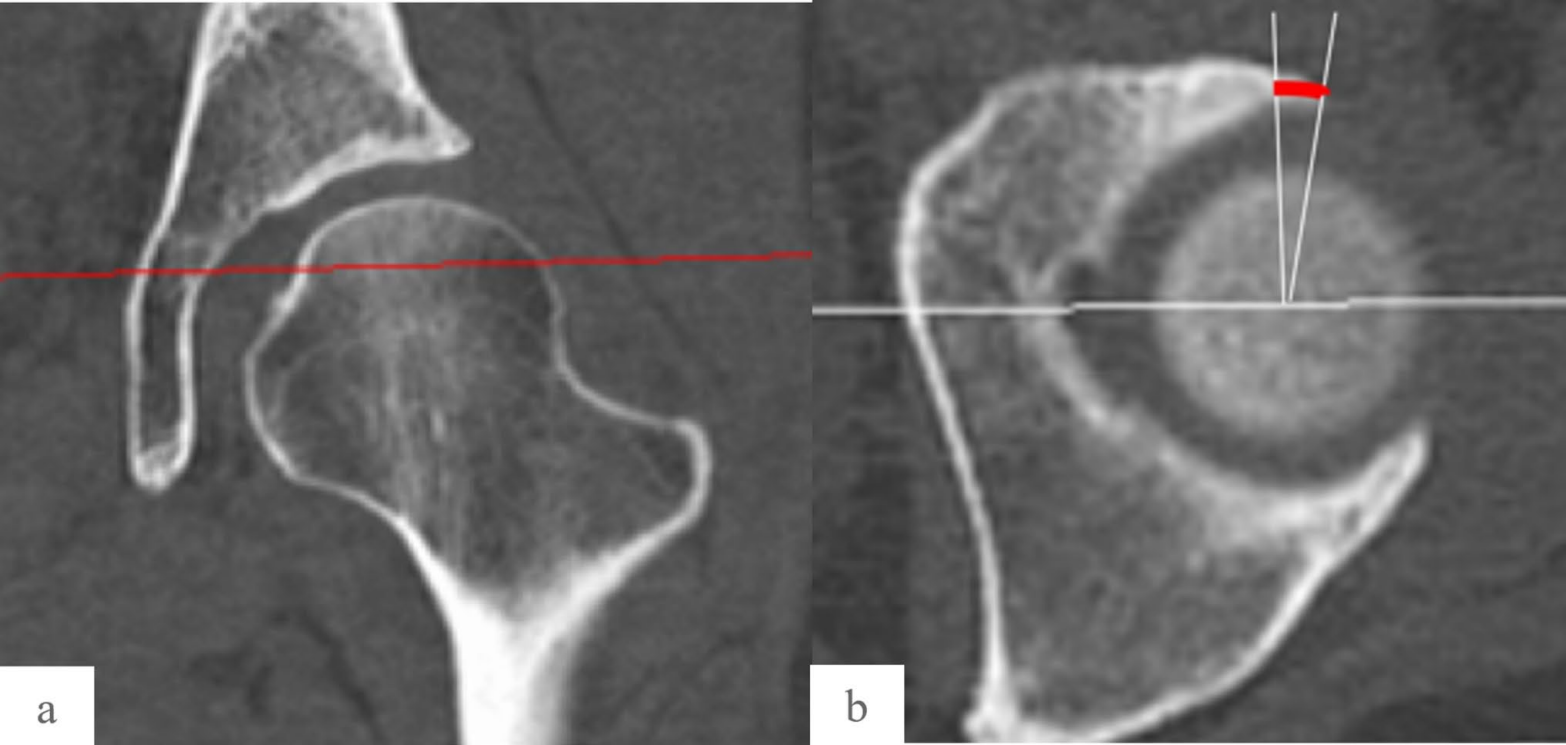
Radiographic indices for evaluating the acetabular version angle. a The plane of measurement is the superior quarter of the femoral head in a computed tomography (CT) coronal image obtained at the level of the central section of the femoral head and bthe acetabular version angle is defined as the angle between a perpendicular line drawn from the center of the femoral head and the anterior rim of the acetabulum on an axial CT image (red curve line)
